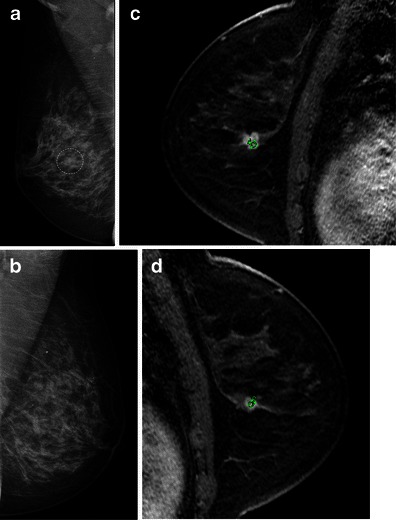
A 49-year-old woman with bilateral ILC. a and b Mammographic oblique views for screening. A dense breast is seen, with a small irregular lesion, indistinct margins in the central right breast (*dotted line*). Core needle biopsy confirmed an ILC and MR exam was requested. Sagittal views, T1 post-contrast, from right (c) and left breasts (d) confirming the right lesion seen on mammography (larger in MR images). However, another lesion is seen in contralateral breast, also with irregular margins and strong enhancement (*circle*). The kinetic curves for index lesion and synchronous contralateral mass were type 3 and 2 curves, respectively, not showed
